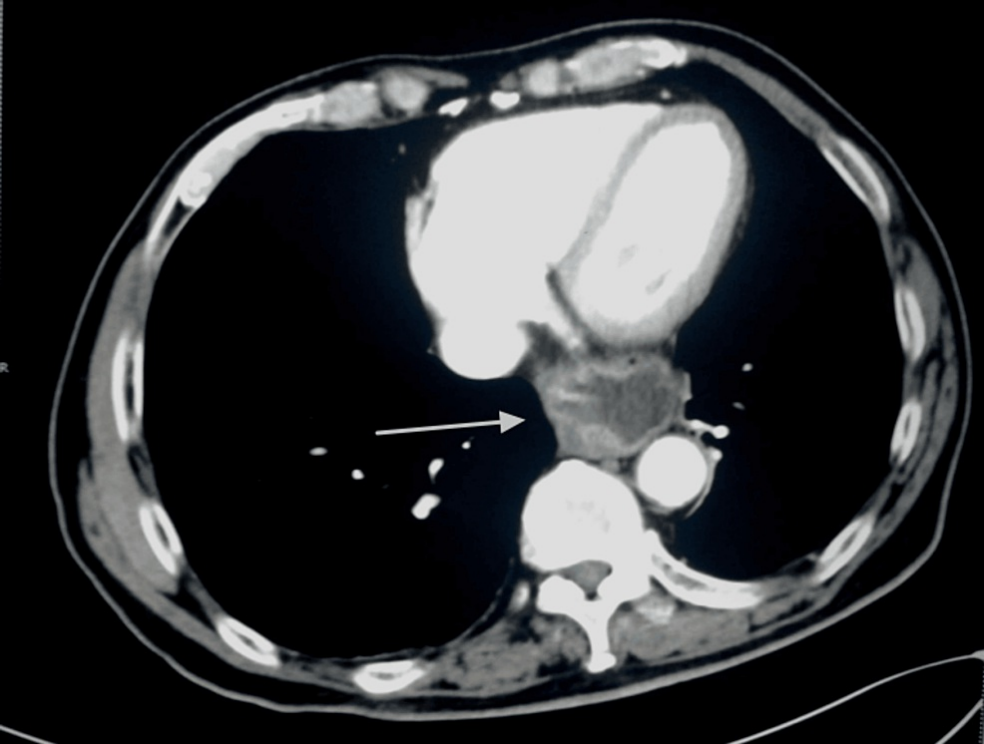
Axial plain CT scan shows diaphragmatic hernia (arrows).
